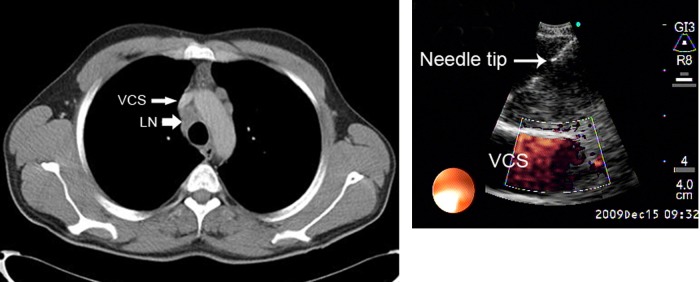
A, Right lower paratracheal lymph node. The tip of the needle is shown with an arrow. The echogenic view under the tip of the needle is an internal echo of the lymph node; B, The correlative CT image of the right lower paratracheal lymph node. Abbreviations: LN; lymph node, VCS; vena cava superior
